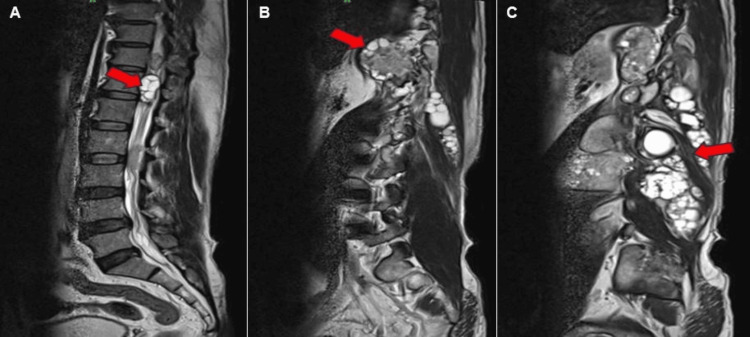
Magnetic resonance imaging of T2-weighted sagittal and parasagittal images of the thoracic and lumbosacral regions. (A) Intraspinal extension of the cysts (red block arrow). (B) Paraspinal muscle involvement with a pleuroperitoneal excursion along with multiple daughter cysts (red block arrow). (C) Multiple daughter cysts (red block arrow) are seen within the mother cyst in right quadratus lumborum and erector spinae muscles.
